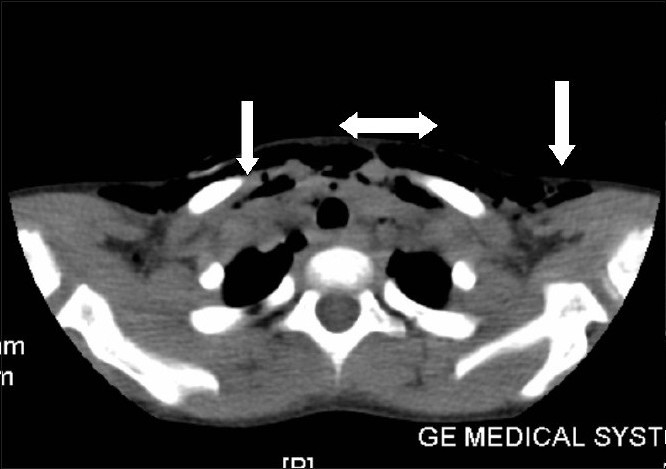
CT picture upper thorax showing subcutaneous emphysema (marked in this picture with white arrows)
